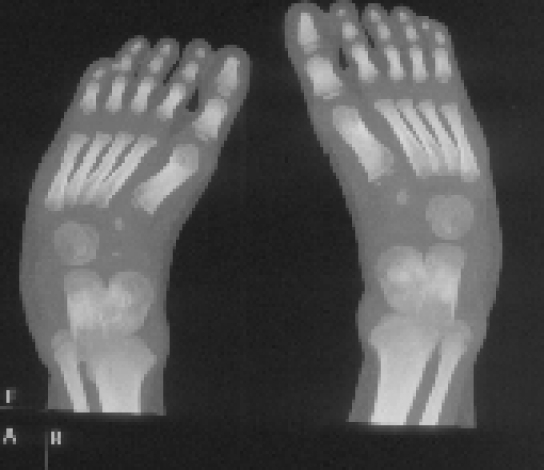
Coronal reformatted CT scan showed a line drawn to the head of the talus and through the body of the talus, makes a Z shape.
